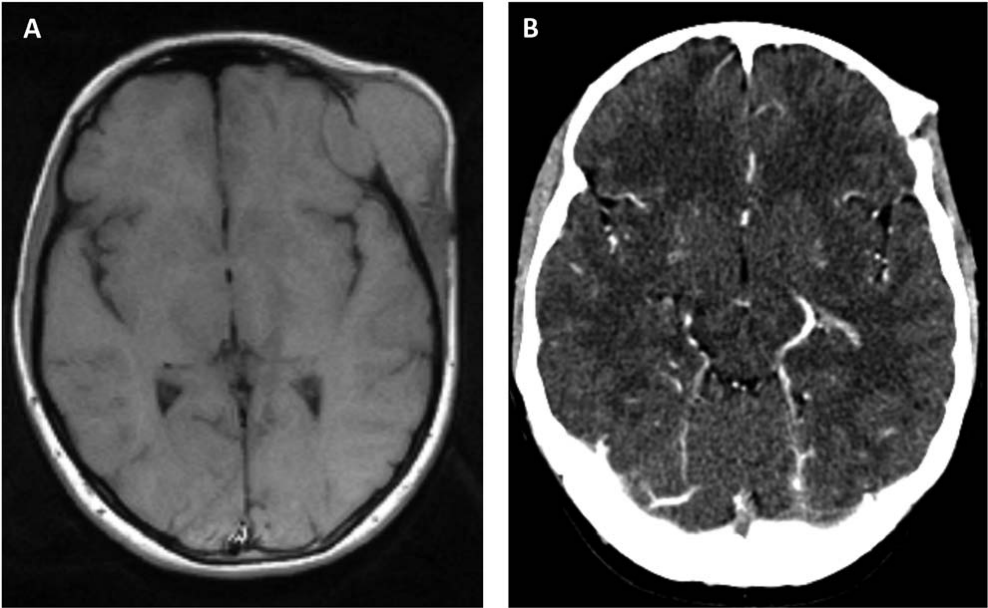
(A) Cranial magnetic resonance imaging shows a large tissue mass (54 mm × 26 mm × 59 mm) in the temporal lobe. (B) Cranial computed tomography shows partial remission (6 mm × 10 mm) after 3 cycles of vincristine, ifosfamide, doxorubicin, and etoposide (VIDE).
